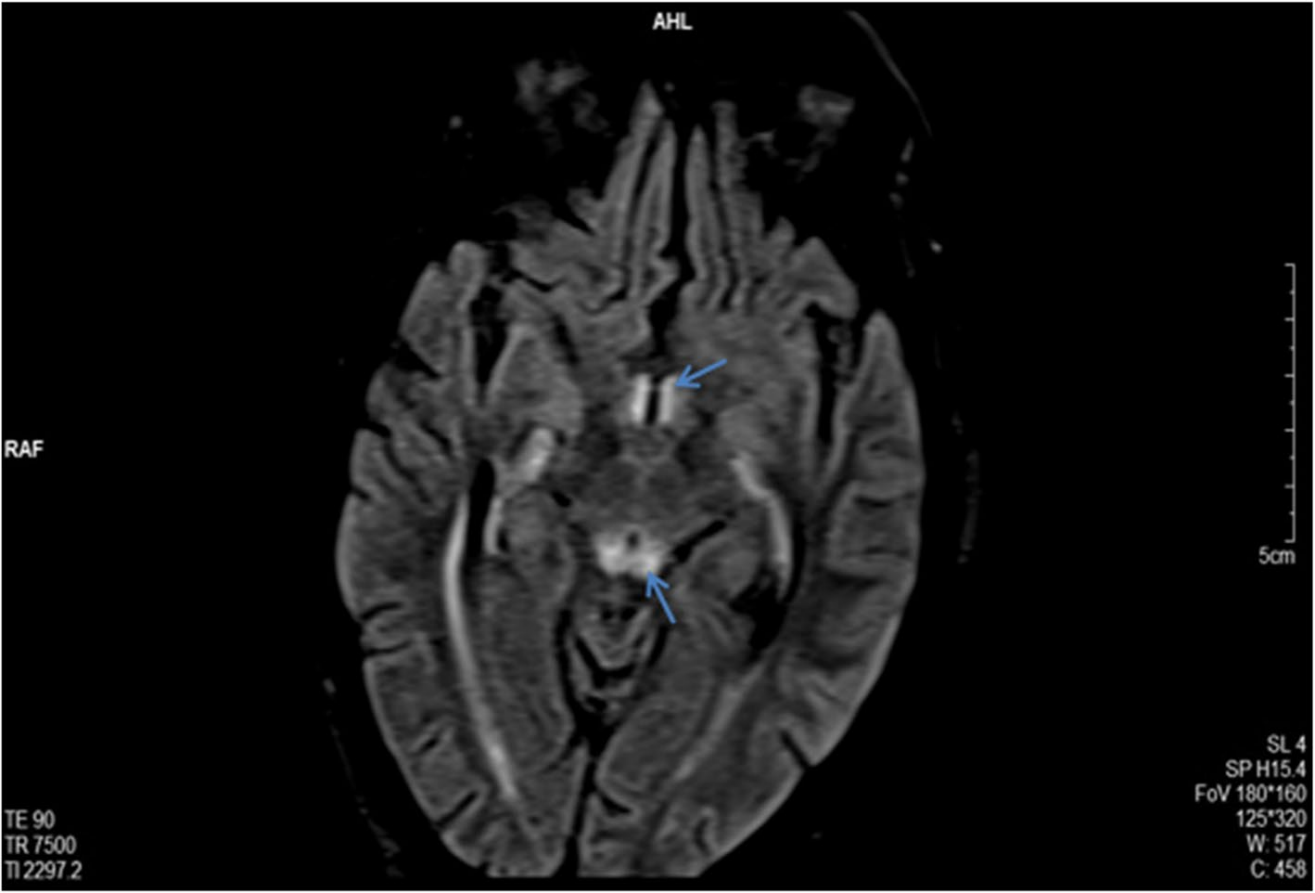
Axial section of Cerebral MRI in FLAIR sequence showing: Hyper signal of the mammary and peri-aqueductal bodies
